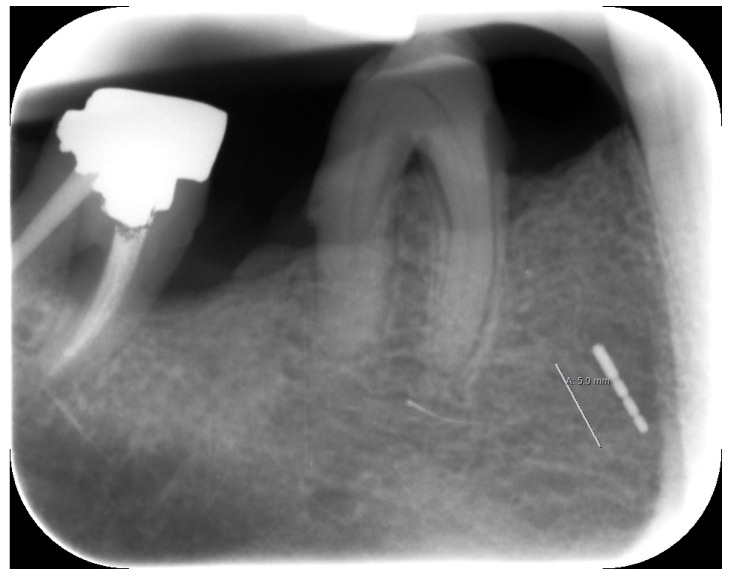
Periapical X-ray with the broken periodontal probe.
